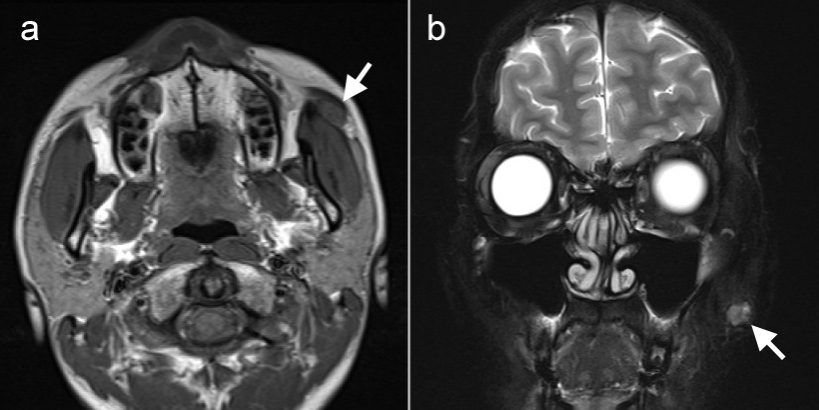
Magnetic resonance imaging of the tumor. The tumor is located on the left masseter muscle (arrows). a. Horizontal plane. The tumor exhibits hypointensity on T1-weighted images. b. Coronal plane. The tumor exhibits slightly higher intensity in T2-weighted imaging with a fat-suppression technique.
